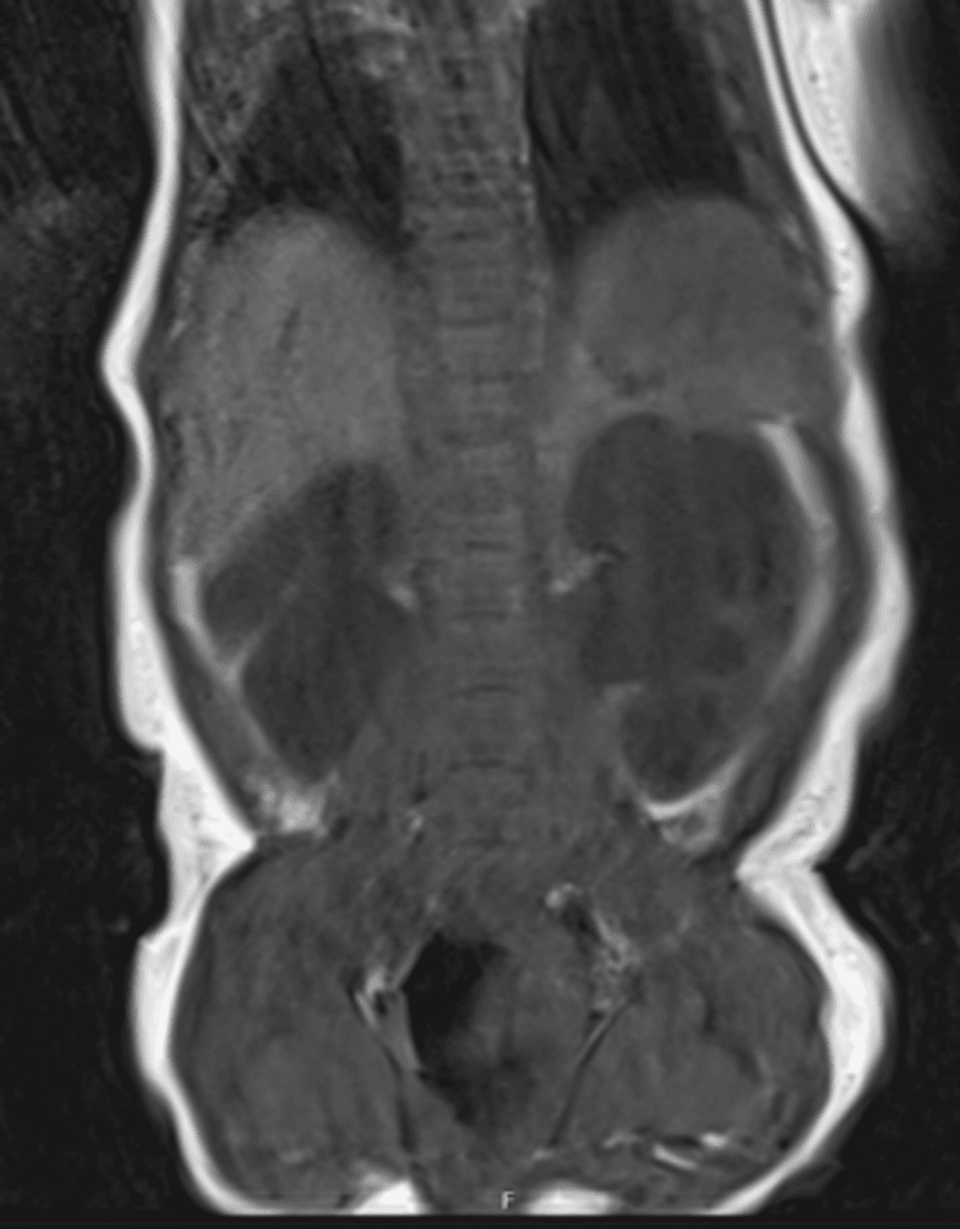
MRI lumbar spine (T1 coronal) showing bilateral hydronephrosis (motion artifact present).
